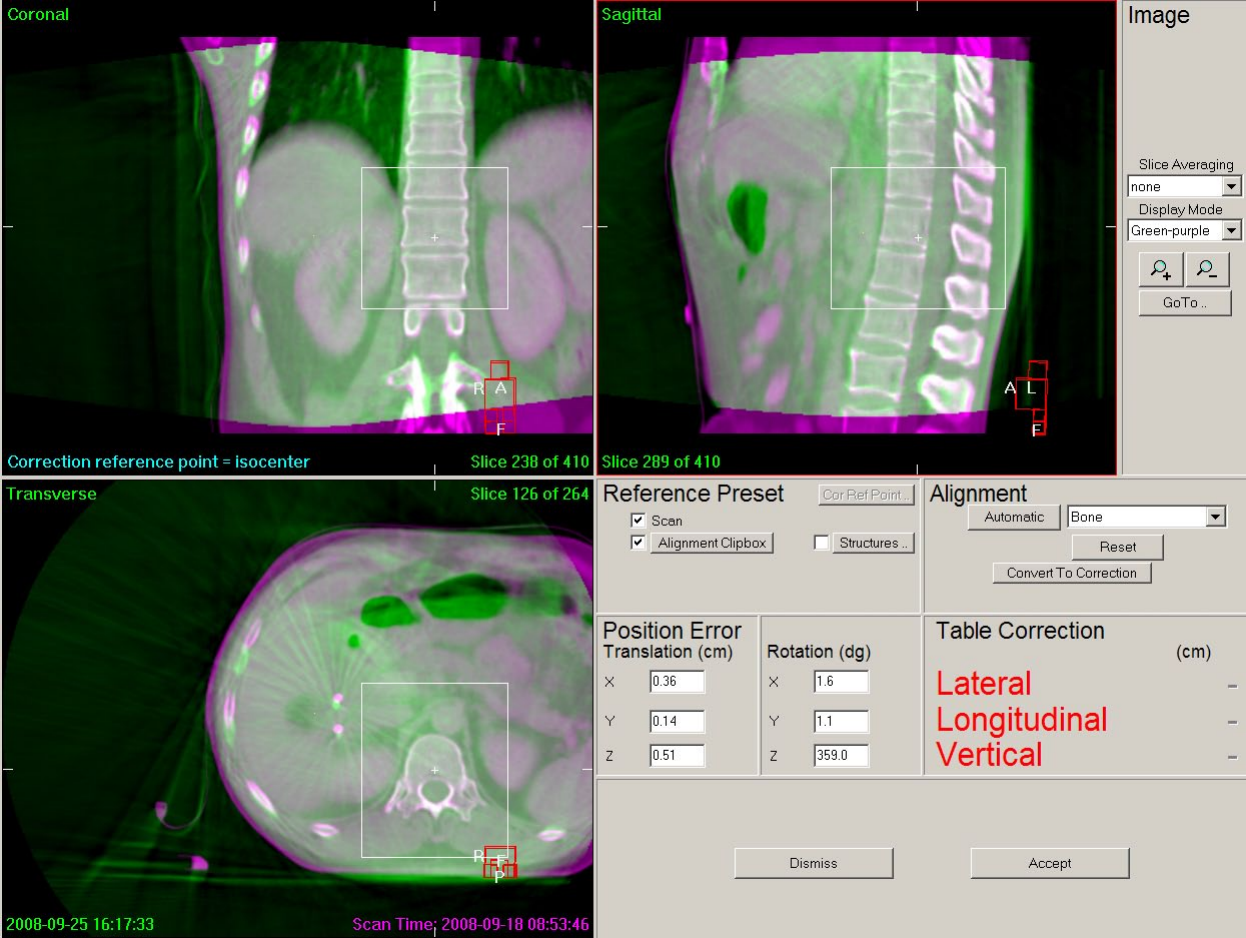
Vertebral body match in which CBCT images are shown in green and reference CT images are shown in purple. The white line is clipbox, which included the vertebral bodies corresponding to the PTV slices. Bones were white when bones from two image sets were completely fit.
